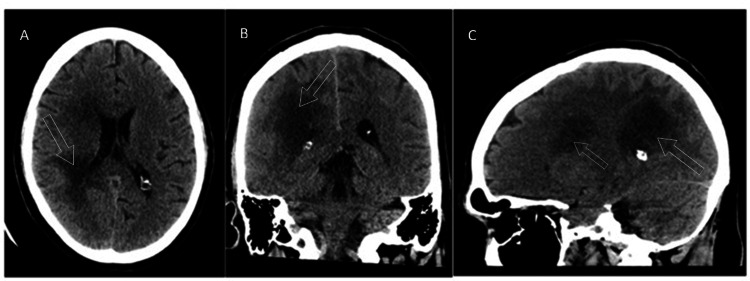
Initial nonenhanced CT Unenhanced CT head showing decreased attenuation of the right cerebral white matter, as designated by the unfilled white arrows. The findings were primarily concentrated within the parietal but also were seen in the occipital, posterior temporal, and frontal white matter within the axial (A), coronal (B), and saggital (C) planes. There was an associated mass effect as suggested by the sulcal and lateral ventricular effacement.
